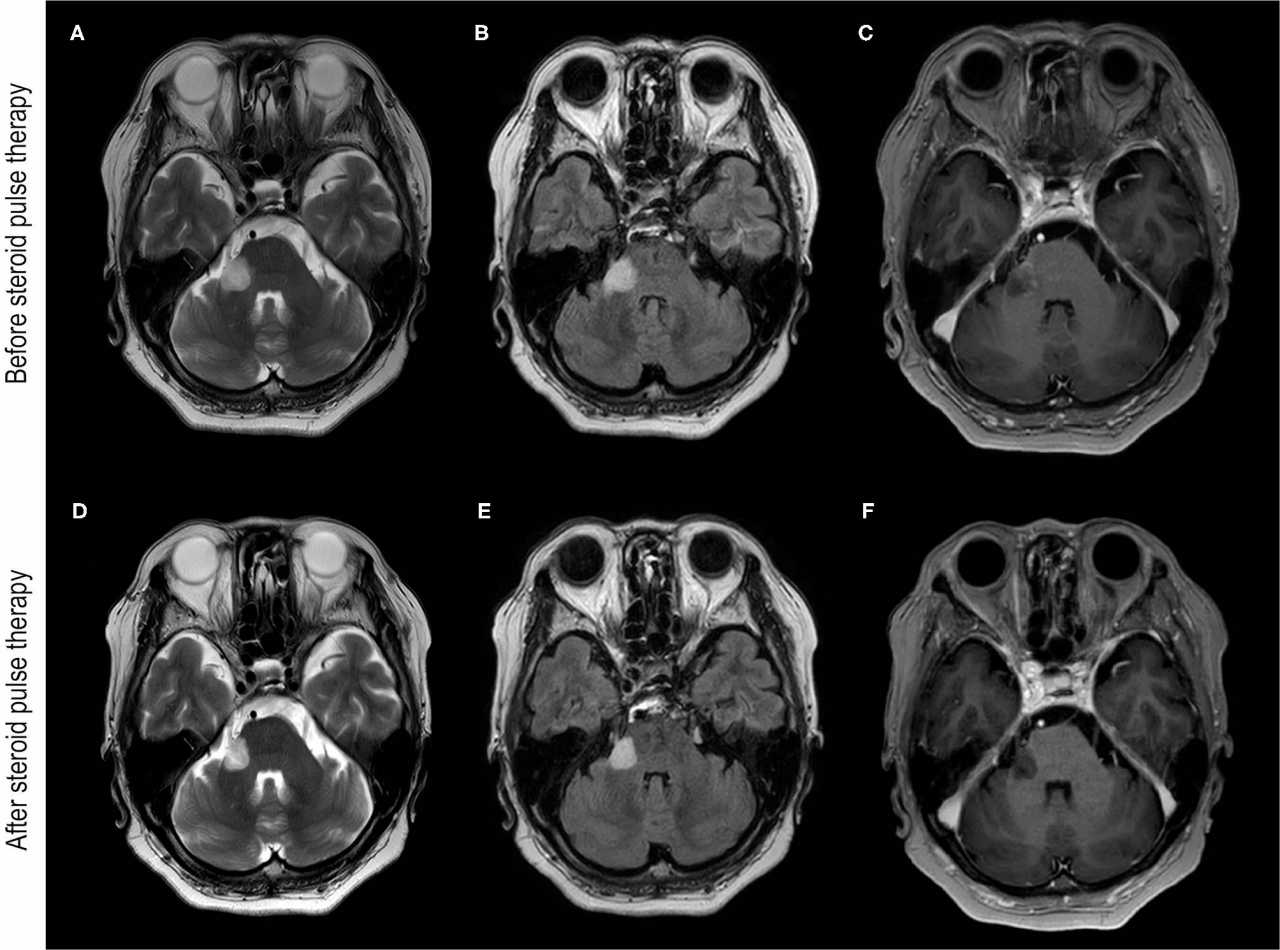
Brain MRI images before and after steroid pulse therapy. (A–C) Were obtained before steroid pulse therapy. Brain MRI of T2-weighted (A) and fluid-attenuated inversion recovery (FLAIR) (B) images showing hyperintensity of the cerebellar peduncle and the root of trigeminal nerve. (C) T1-weighted brain MRI with gadolinium enhancement (T1Gd) showing enhancement around the lesion. (D–F) were obtained after steroid pulse therapy. Brain MRI of T2-weighted (D) and FLAIR images (E) showing the reduction in the hyperintensity lesion of the cerebellar peduncle. (F) T1Gd brain MRI showing no gadolinium enhancement after steroid therapy.
